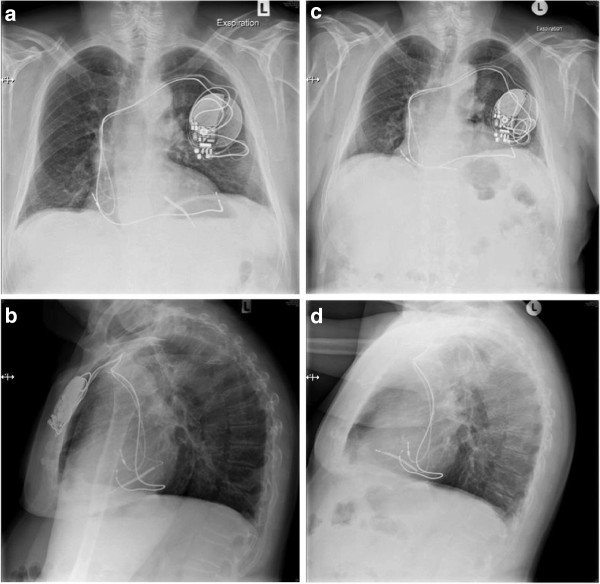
Chest x-ray in the anterior and lateral view after the original CRT-ICD implantation (2 a-b) and after revision of the ICD lead (2 c-d). Atrial and CS electrodes remained unchanged. During revision the malplaced ICD electrode was removed and replaced in the RV. The chest x-ray after first implant was graded normal by routine judgement. In retrospective, the curve at the atrial level and the posterior orientation of the ICD lead may indicate passage of the foramen ovale and misplacement in the LV.
